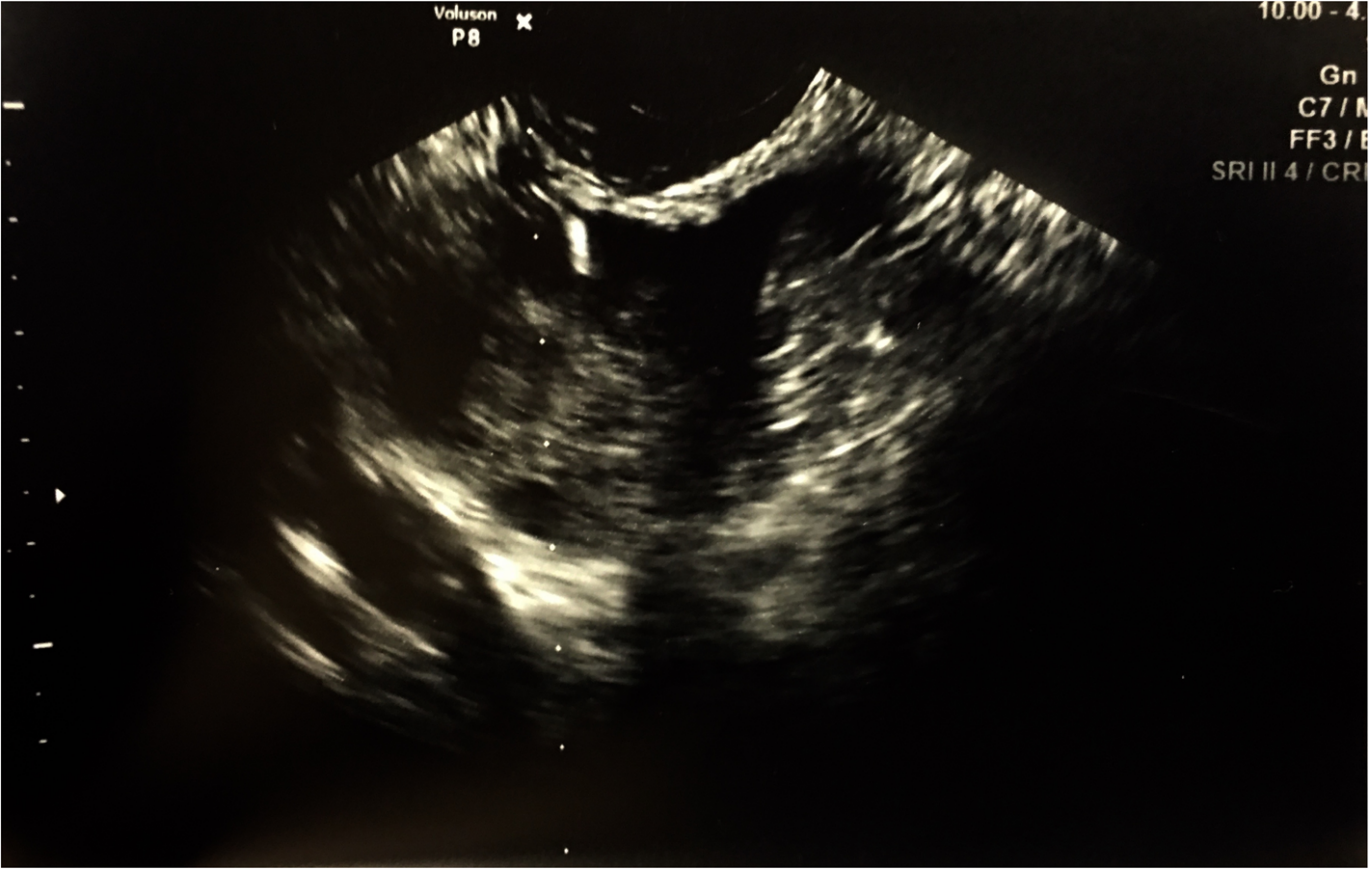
Tip of aspiration needle inside a follicle under ultrasound
